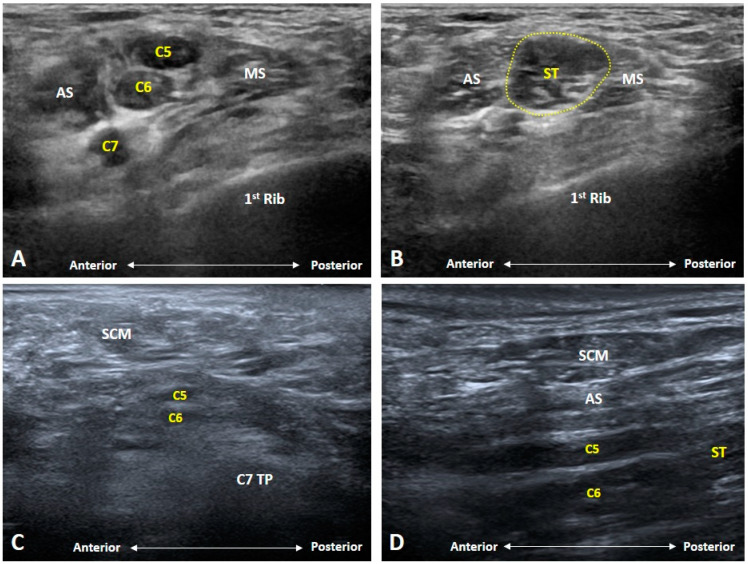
Ultrasound imaging of radiation-induced brachial plexopathy. Nerve fascicles of the brachial plexus at the root (A) and trunk (yellow dashed area) (B) levels appear enlarged with thickened epineurium. The muscles surrounding the neck are atrophic and fibrotic in the short-axis (C) and long-axis (D) views. AS: anterior scalene muscle; MS: middle scalene muscle; SCM: sternocleidomastoid muscle; ST: superior trunk.
